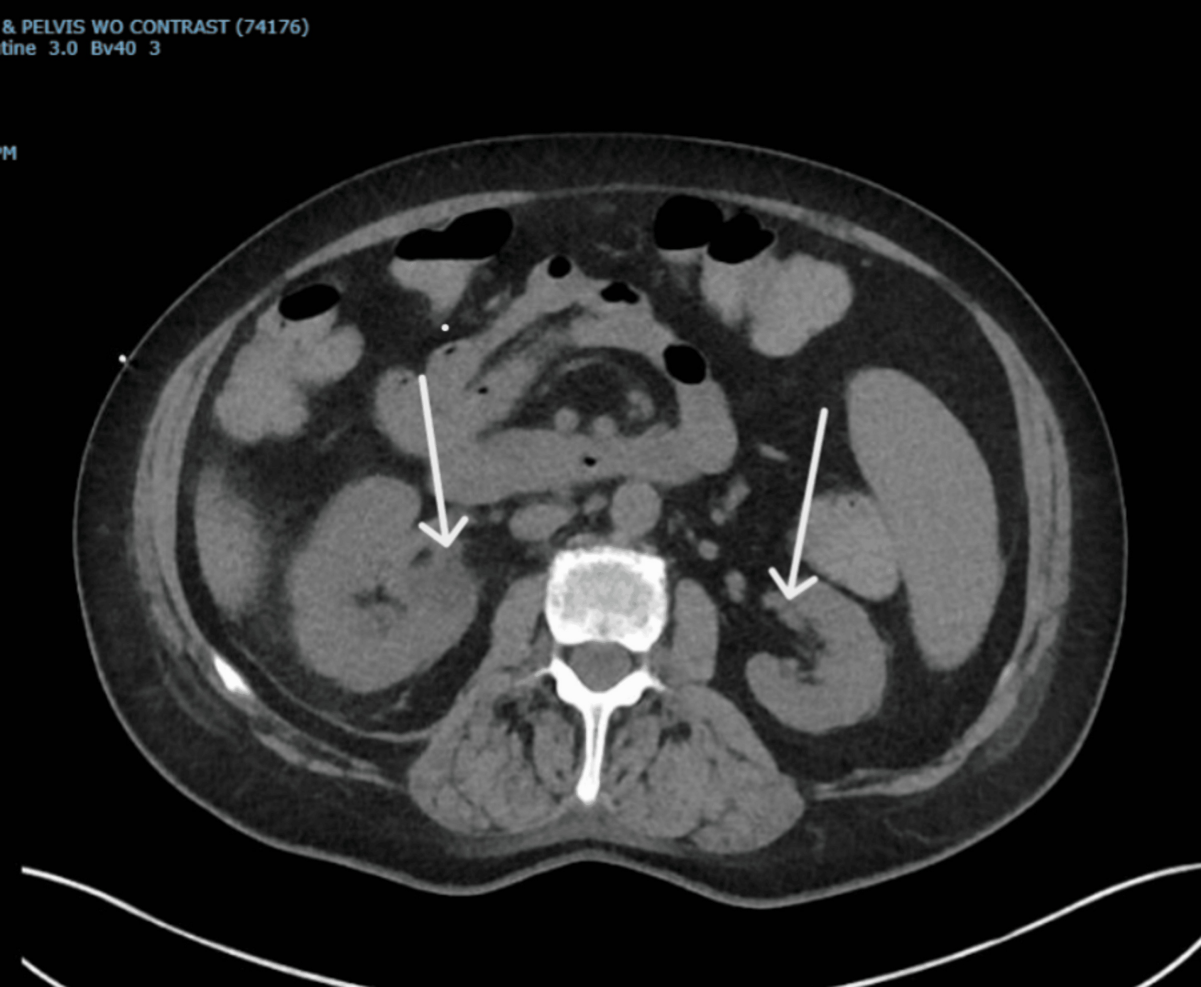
CT scan of the abdomen and pelvis without contrast. Bilateral adrenal enlargement, more pronounced on the right side, showing high attenuation areas (hyperdense) consistent with an adrenal hematoma (arrows).
